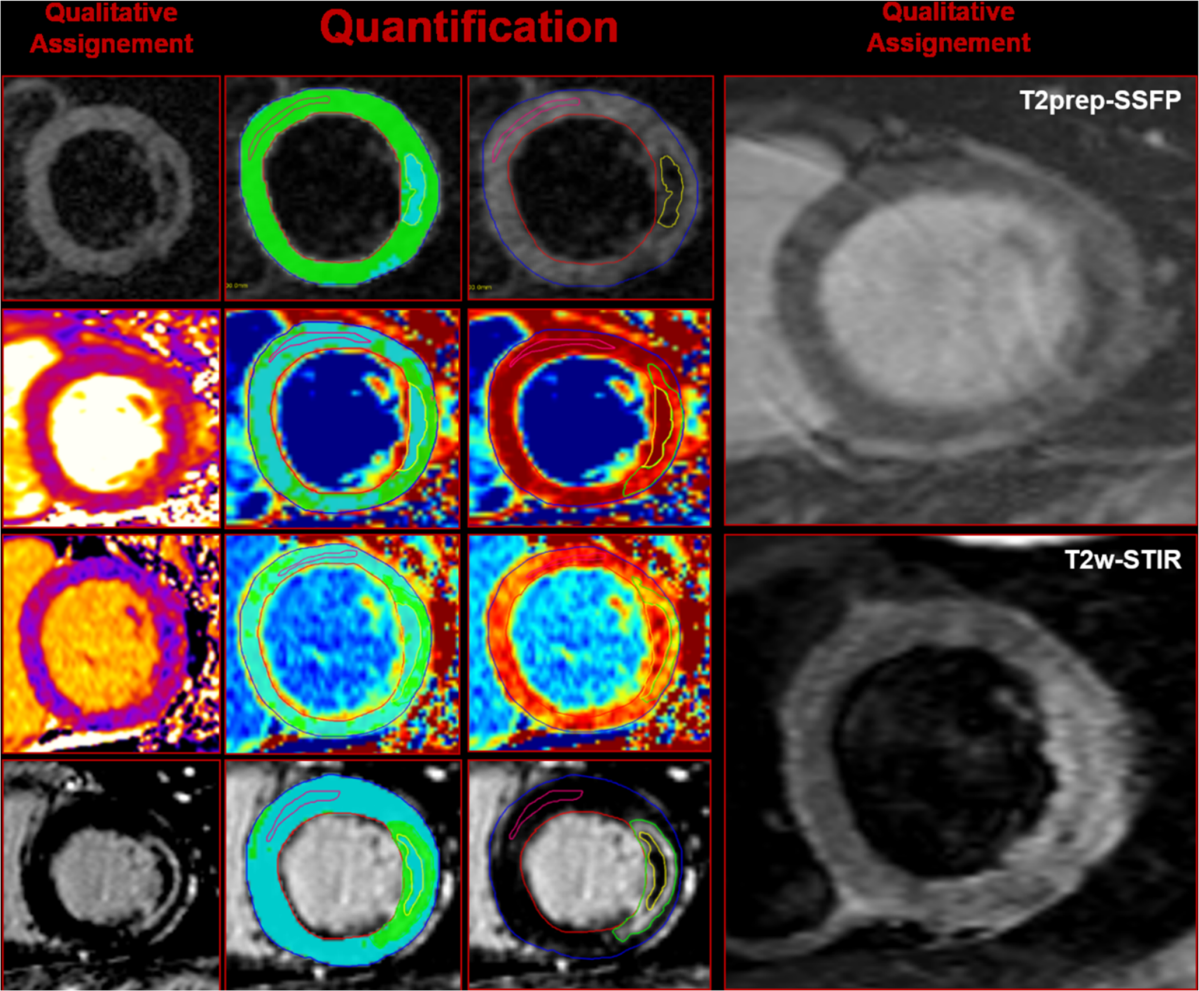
Qualitative and quantitative assessments of the target slices. For T2*w, T2/T1 maps, and LGE, the analysis started with the qualitative evaluation (first column) to continue then with the quantitative assessment (2nd and 3rd columns). For T2w-STIR and T2prep-SSFP, only the qualitative assessment is carried out (4th column). LGE and MVO quantifications were not included in the comparative analysis with T2*w for IMH. *Abbreviations*: T2*w, T2*-weighted; T2 map, T2 mapping; T1 map, T1 mapping; IMH, intramyocardial haemorrhage; T2prep-SSFP, T2 preparation steady-state-free precession; T2w-STIR, T2-weighted short-TI inversion recovery; MVO, microvascular obstruction; LGE, late gadolinium enhancement
